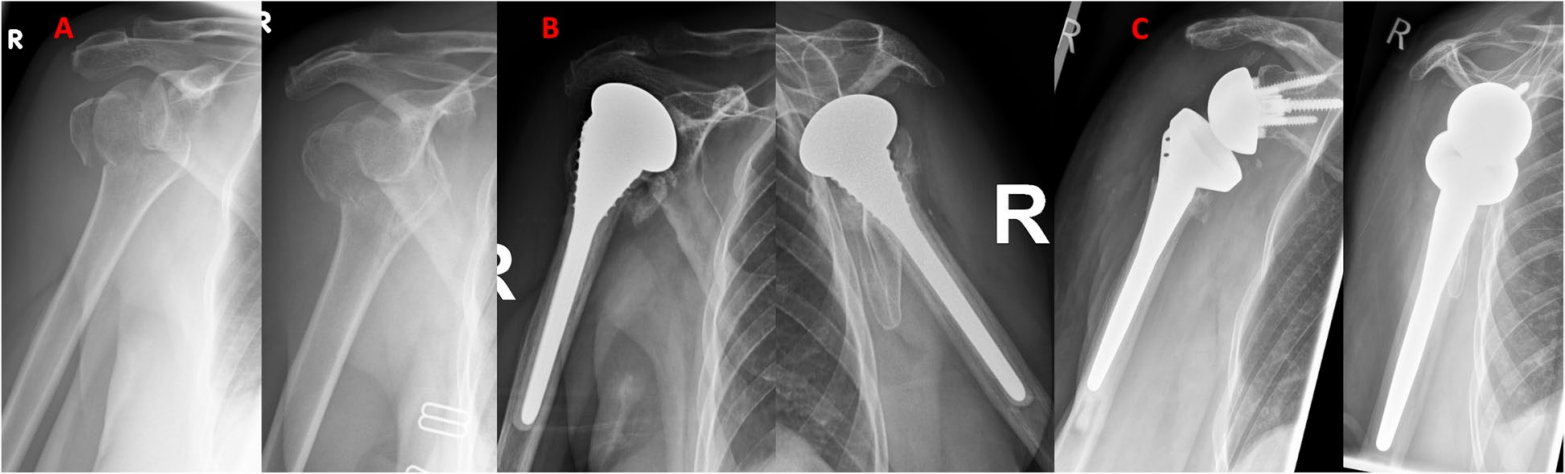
Radiographs obtained during the course of reverse total shoulder arthroplasty revision procedure for failed hemiarthroplasty after proximal humerus fracture. A A 66-year-old patient from our cohort fell on to her right shoulder at home, hemiarthroplasty was indicated in this case. B Prosthesis failure due to tuberosity and rotator cuff insufficiency with postoperative persistent pain and stiffness. C Implantation of a reverse total shoulder endoprosthesis was performed
